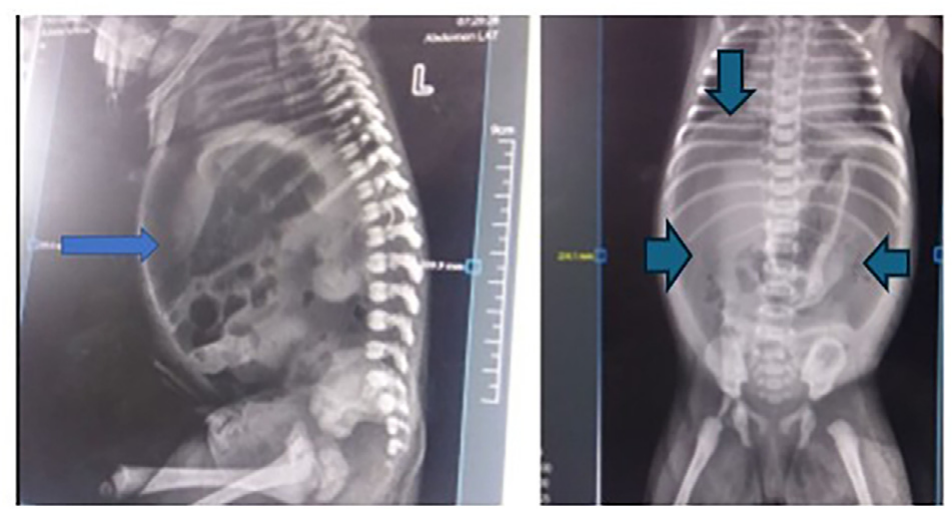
AP and lateral x-ray showing significant pneumoperitoneum.
